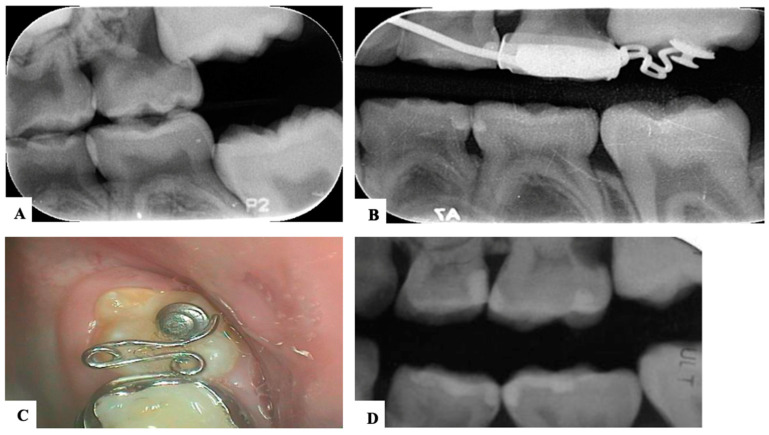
Ectopic maxillary first permanent molar with Halterman appliance to tip the first molar distally in a 5-year-old girl. (A). Pre-treatment radiograph showing maxillary left ectopic permanent molar. (B). Bitewing radiograph during appliance treatment. (C). Clinical photograph of the Halterman appliance 6 weeks later. (D). Post-treatment radiograph showing satisfactory eruption of the permanent molar. (Courtesy of Dr. Abu-Hussein M, permission granted.)
